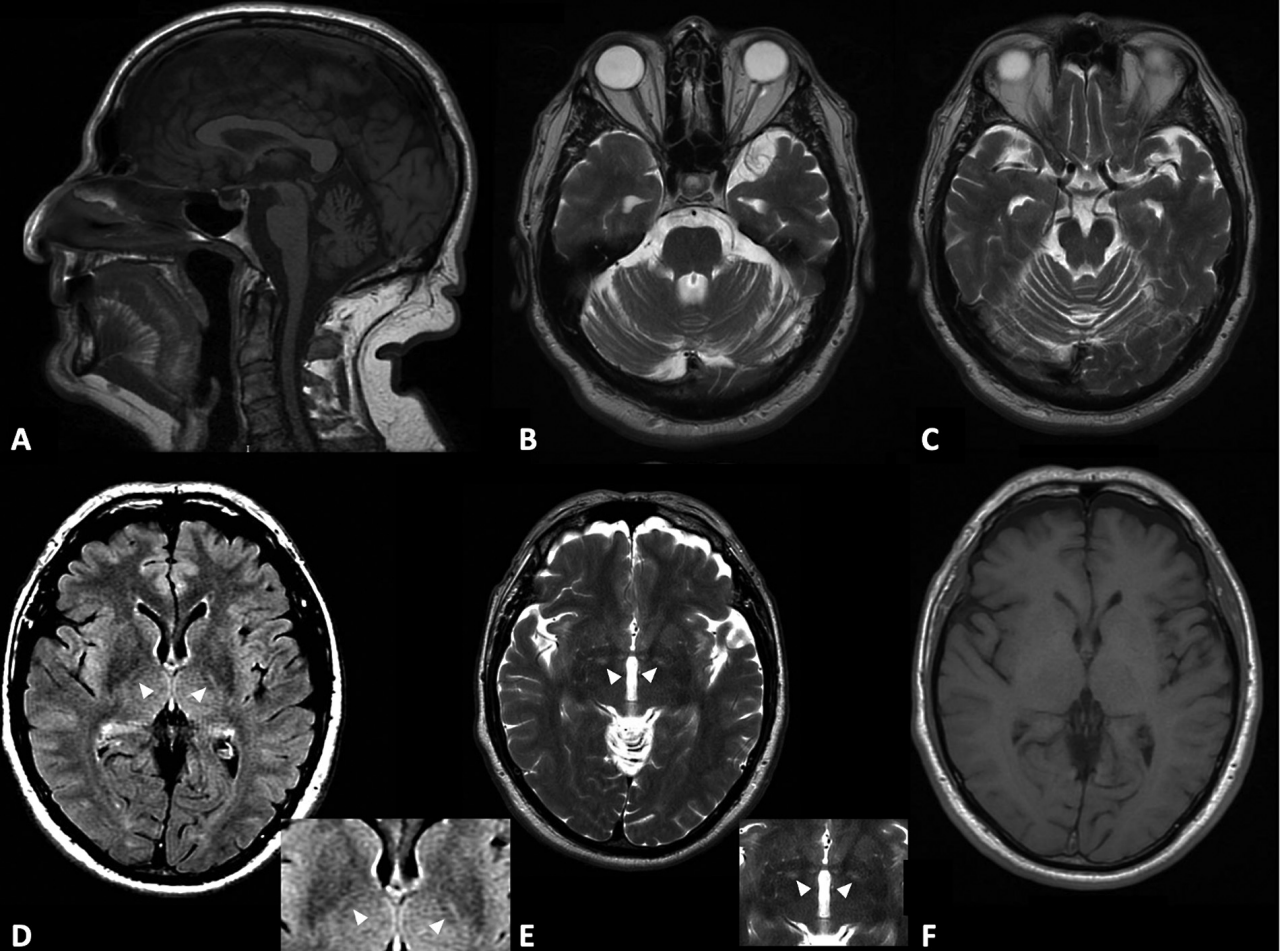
T2 and FLAIR hyperintensity in a patient with Machado-Joseph disease (SCA3). Cerebellar atrophy is shown in sagittal T1 (
